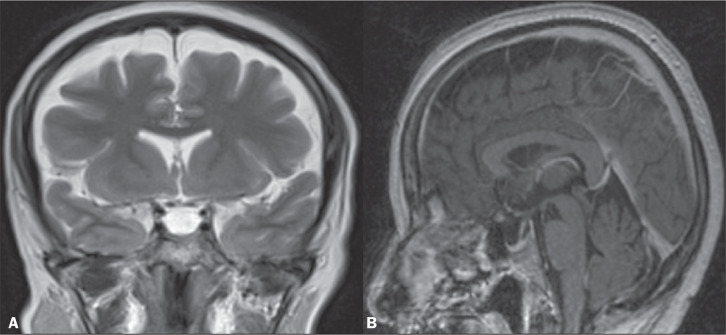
Coronal T2-weighted MRI scan (A) and contrast-enhanced sagittal
